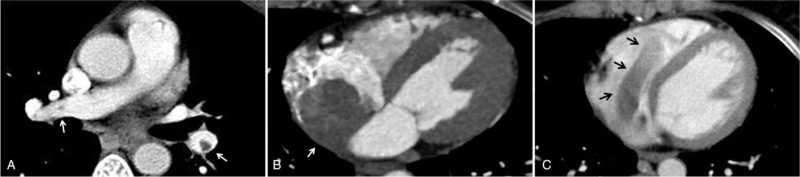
(A) Computed tomography angiogram showing multiple thrombi (arrow) in the right pulmonary artery and left interlobar artery. (B) Arrow indicates a large right atrial thrombus extending into the inferior vena cava. (C) Arrow indicates right atrial thrombus protruding into the right ventricle.
